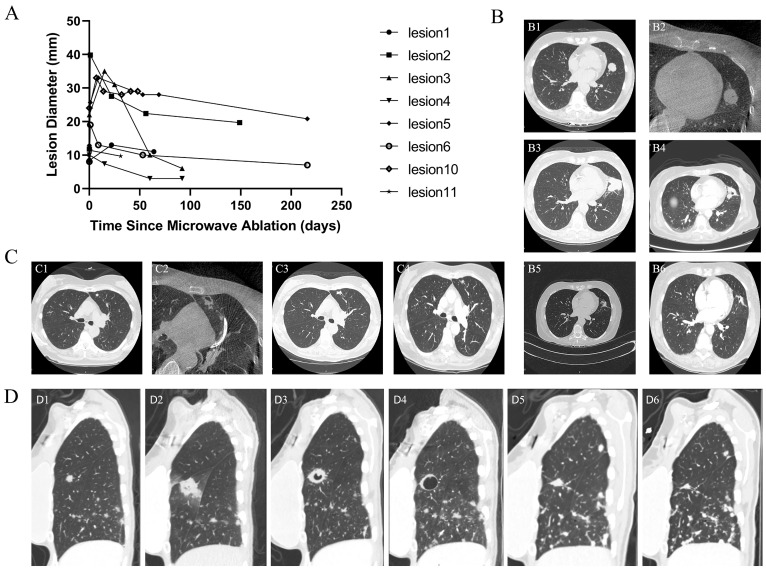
Early radiologic evolution of metastatic pulmonary nodules following ssRAB-guided microwave ablation. (A) Size-change curves of eight metastatic pulmonary nodules following MWA. (B) Serial chest CT images from Case 3- LB5 lesion. (B1) Pre-MWA baseline; (B2) Immediately post-MWA; (B3) 15 days post-MWA; (B4) 25 days post-MWA; (B5) 60 days post-MWA; (B6) 110 days post-MWA. (C) Serial chest CT images from Case 3- LB3 lesion. (C1) Pre-MWA baseline; (C2) Immediately post-MWA; (C3) 15 days post-MWA; (C4) 110 days post-MWA. (D) Serial chest CT images from Case 4. (D1) Pre-MWA baseline; (D2) 1 day post-MWA; (D3) 53 days post-MWA; (D4) 125 days post-MWA; (D5) 216 days post-MWA; (D6) 283 days post-MWA.
